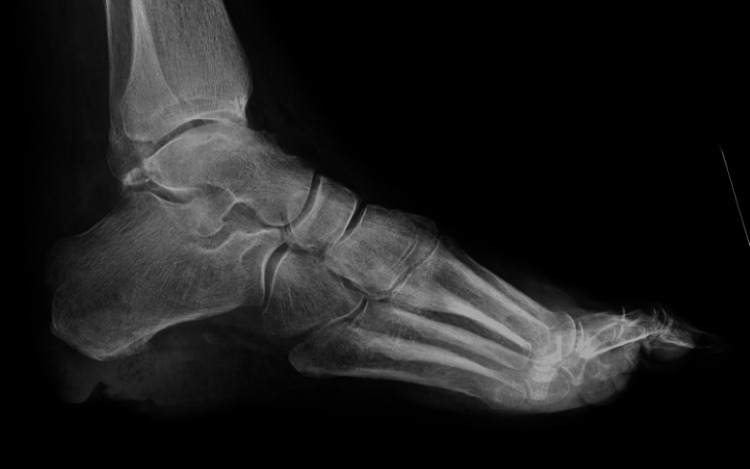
Plain film radiograph of the foot. This patient's X-ray image demonstrated sclerotic changes to the plantar calcaneus without signs of osteomyelitis.
